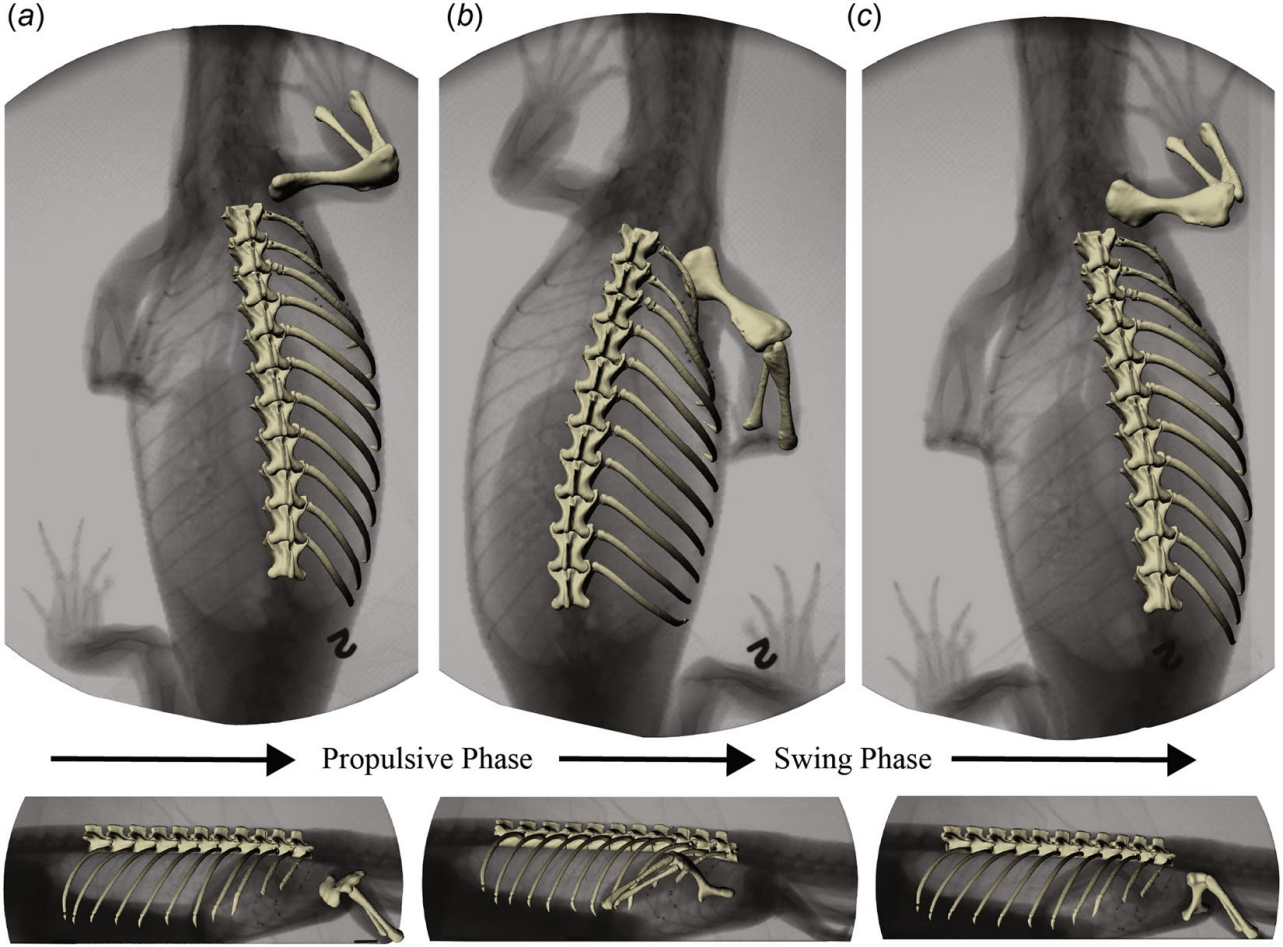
Definition of locomotor stride. XROMM animations were created over the full course of a locomotor stride in both species (*V. exanthematicus* depicted only). Dorsal view (top) and lateral view (bottom) depicting the axial elements, as well as the ipsilateral humerus, radius, and ulna superimposed on the corresponding X-rays. Axial kinematic data were collected for one full cycle starting and ending when the right front limb contacts the treadmill (a). The propulsive phase continues until the limb breaks contact with the treadmill (b), starting the swing phase which continues until the next footfall (c). Stride durations were 1.85 seconds (sav01), 1.56 seconds (sav02), 1.5 seconds (sav03), 1.5 seconds (teg03), 2.83 (te05), and 3.25 seconds (tegu06).
